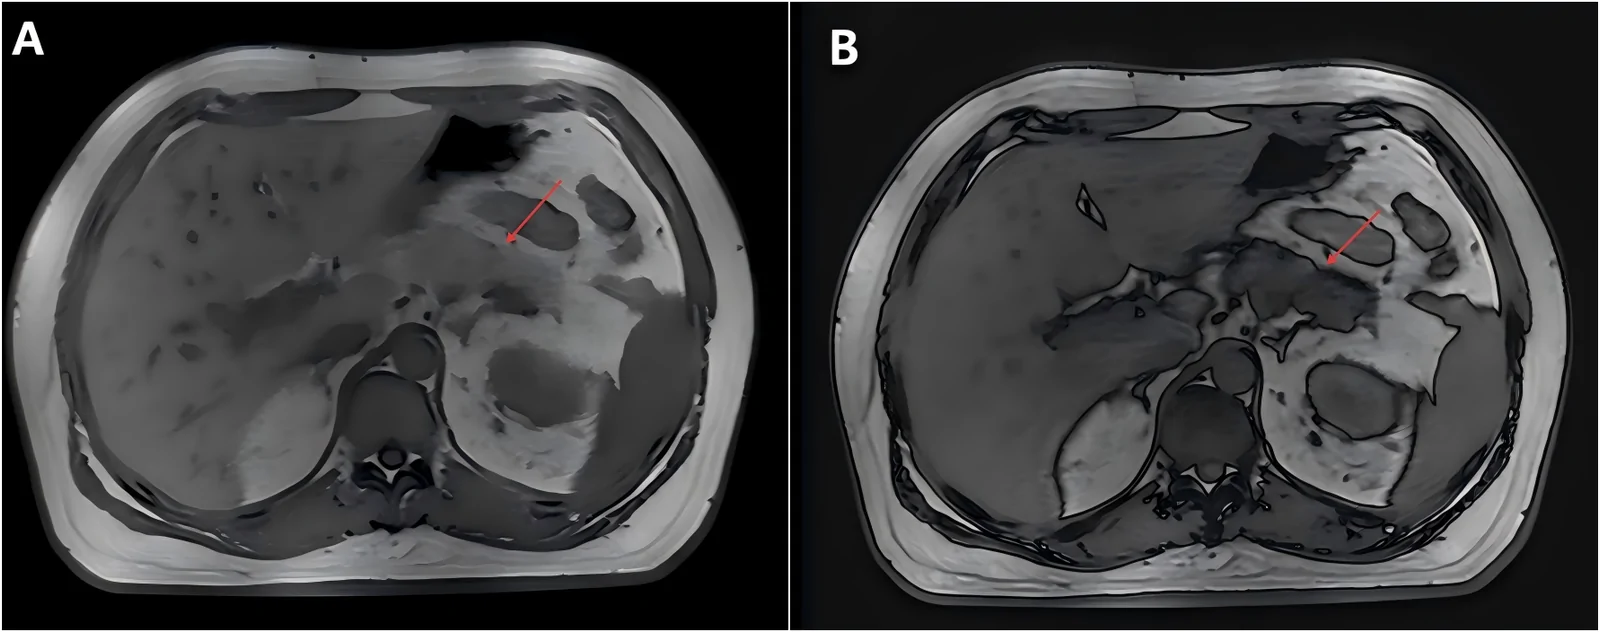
MRI of the patient's pancreas.
